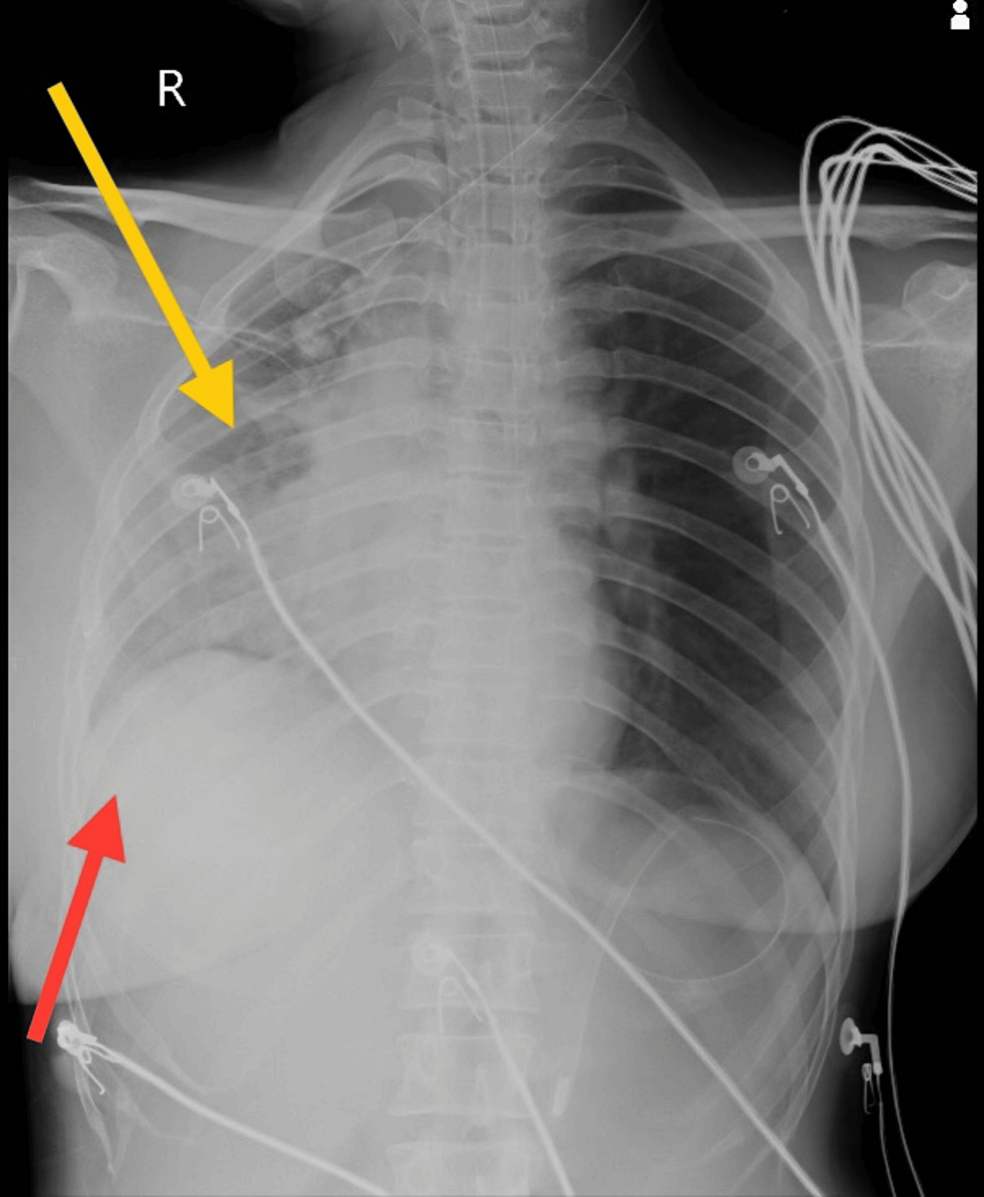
Chest X-ray in the anteroposterior (AP) view Yellow arrow depicts haziness on the right side of the chest with heterogenous opacity. Red arrow depicts elevated diaphragm with blunting of the costophrenic angle.
